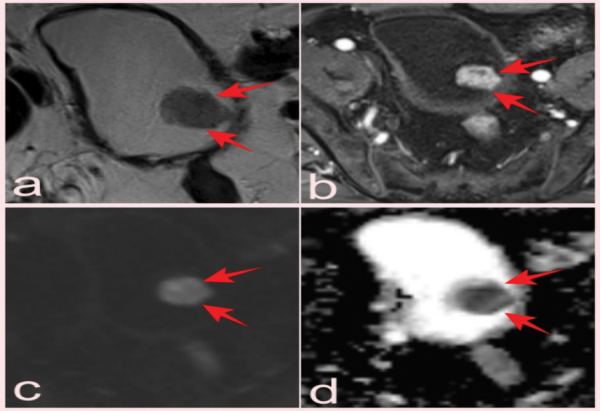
T2-WI (a), DCE images (b), DWI (c), and (d) ADC map of an incidental bladder lesion reported as VI-RADS 3 but pathologically diagnosed as high-grade pT2 small-cell neuroendocrine carcinoma on prostate mpMRI performed after elevated PSA in a 69-year-old patient (arrows).
